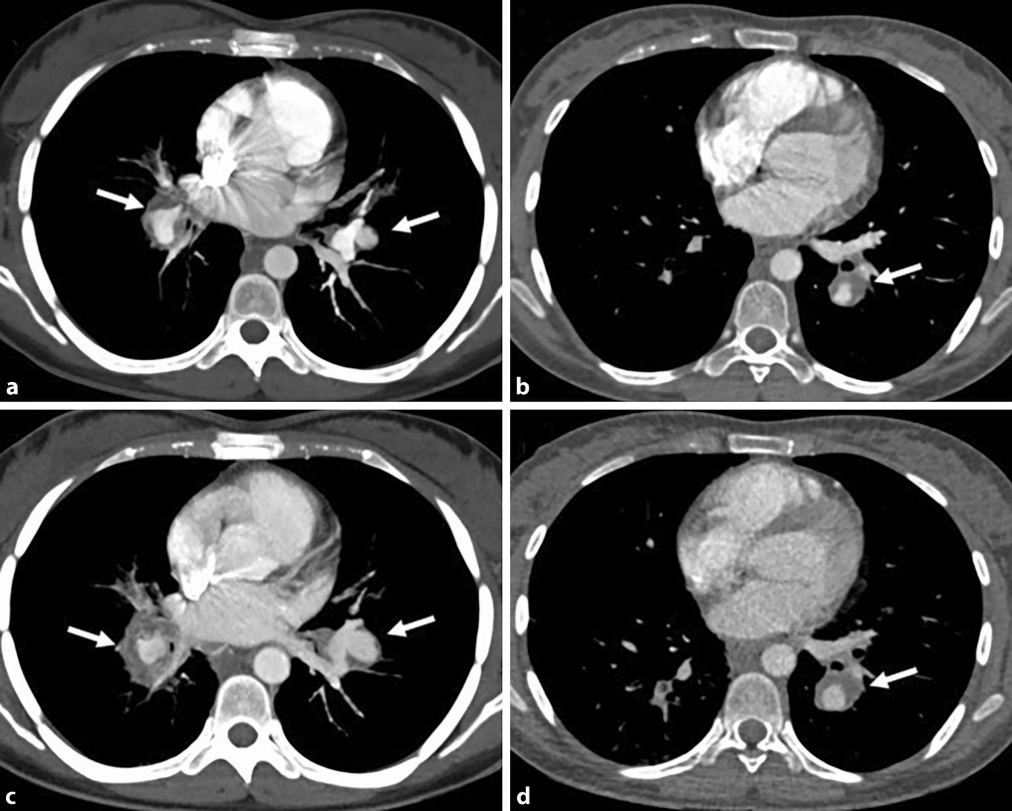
CT pulmonary angiography at presentation (a and b) and CT follow-up after 3 weeks (c and d) showing a progression of the pulmonary artery aneurysms on both sides (*arrows*) (on the right side, from 2 to 2.6 cm, on the left side, from 1.4 to 1.9 cm)
